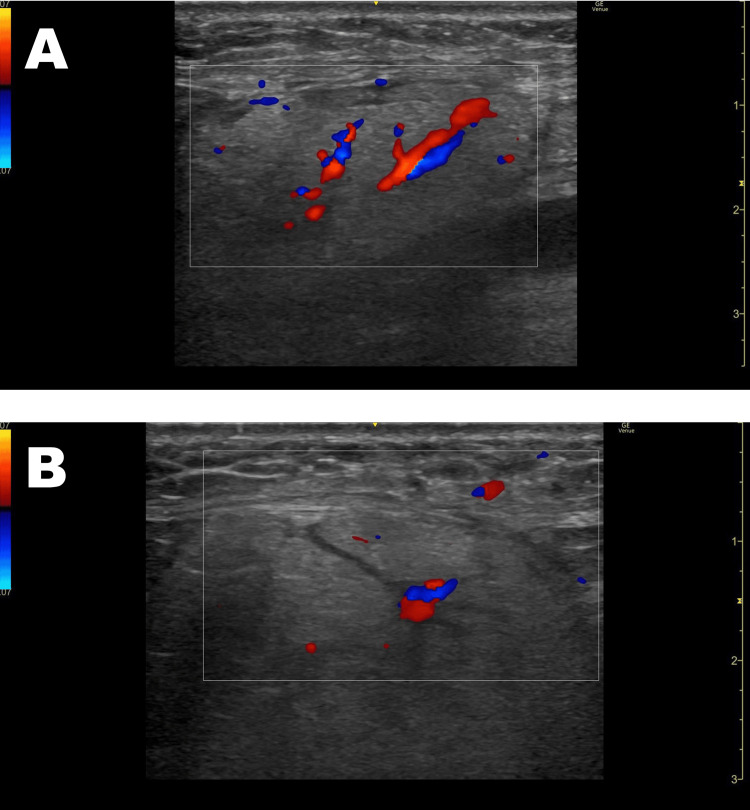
Neck color Doppler ultrasound findings (HOD1). (A) Right submandibular gland. (B) Left submandibular gland Bilateral submandibular gland enlargement with tubular structures suggestive of salivary duct involvement is observed. Color Doppler imaging shows increased blood flow in the central region of the submandibular glands. HOD1: hospital day 1
